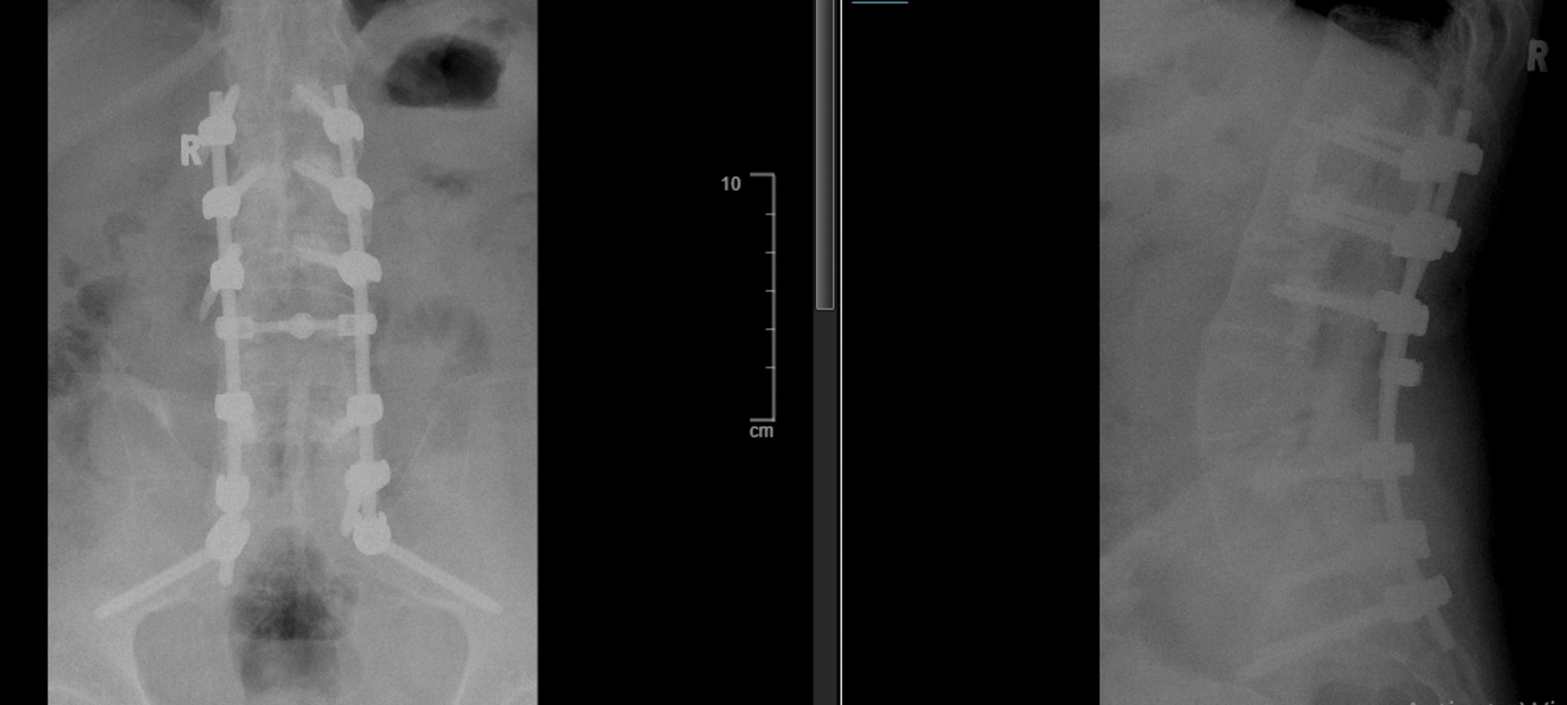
Anteroposterior and lateral view of a lumbosacral X-ray postoperatively: PSIF from L1 to S2 with cement augmentation PSIF: posterior spinal instrumentation and fusion
